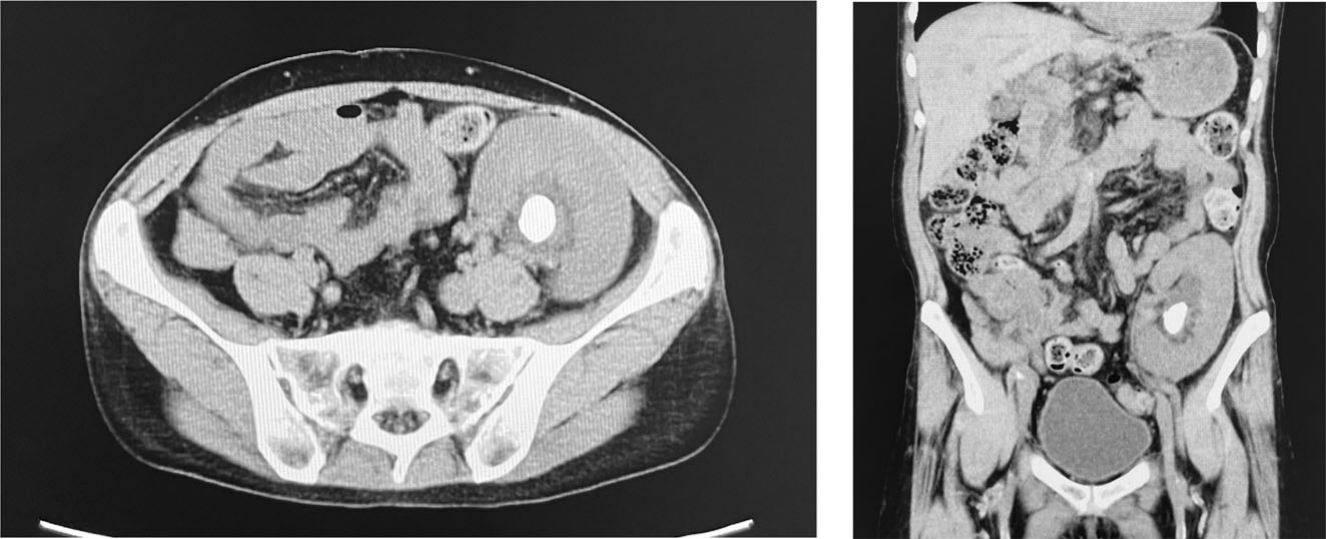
Preoperative CT images of a kidney allograft stone. The present case pertained to a simultaneous pancreas–kidney transplant recipient, with the transplanted kidney placed in the left iliac fossa. The transplanted renal pelvis showed a stone measuring 23 mm in its longest diameter, with an average CT value of 856 Hounsfield Units (maximum, 1092; minimum, 504).
